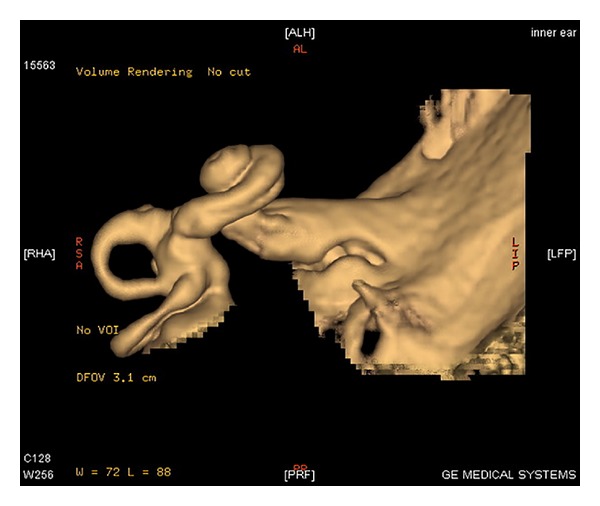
MRI scan with 3D reconstruction of inner ear showing cochlear anomaly in which cochlea shows 1 and 1/2 turns.
